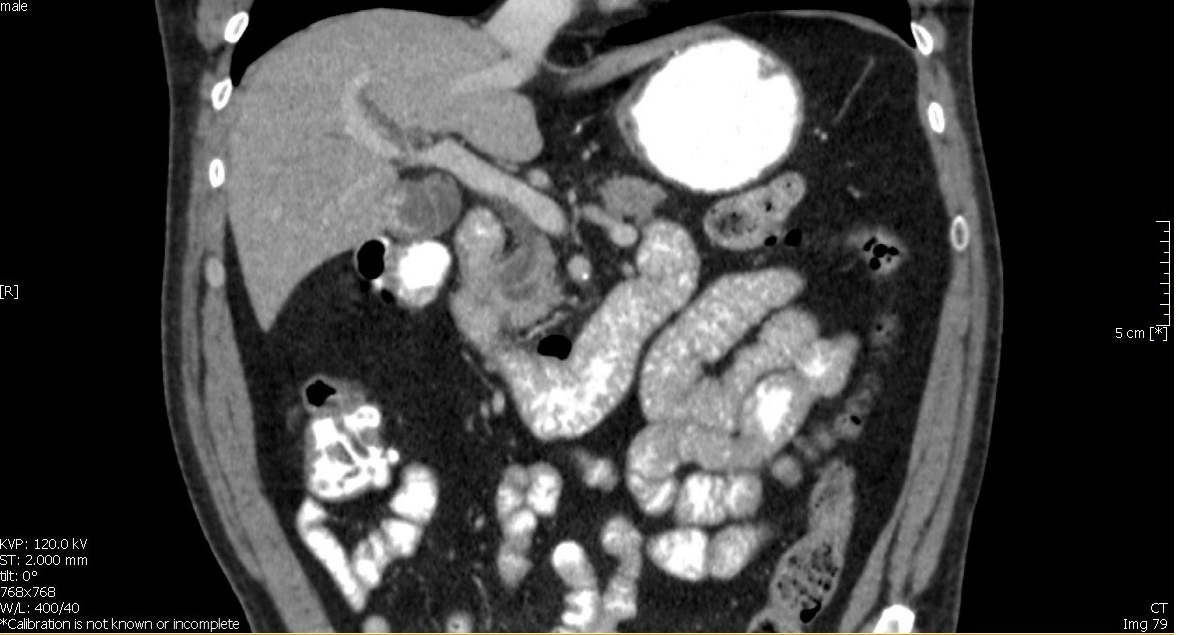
Abdominal Computed Tomography showed the double duct sign.
